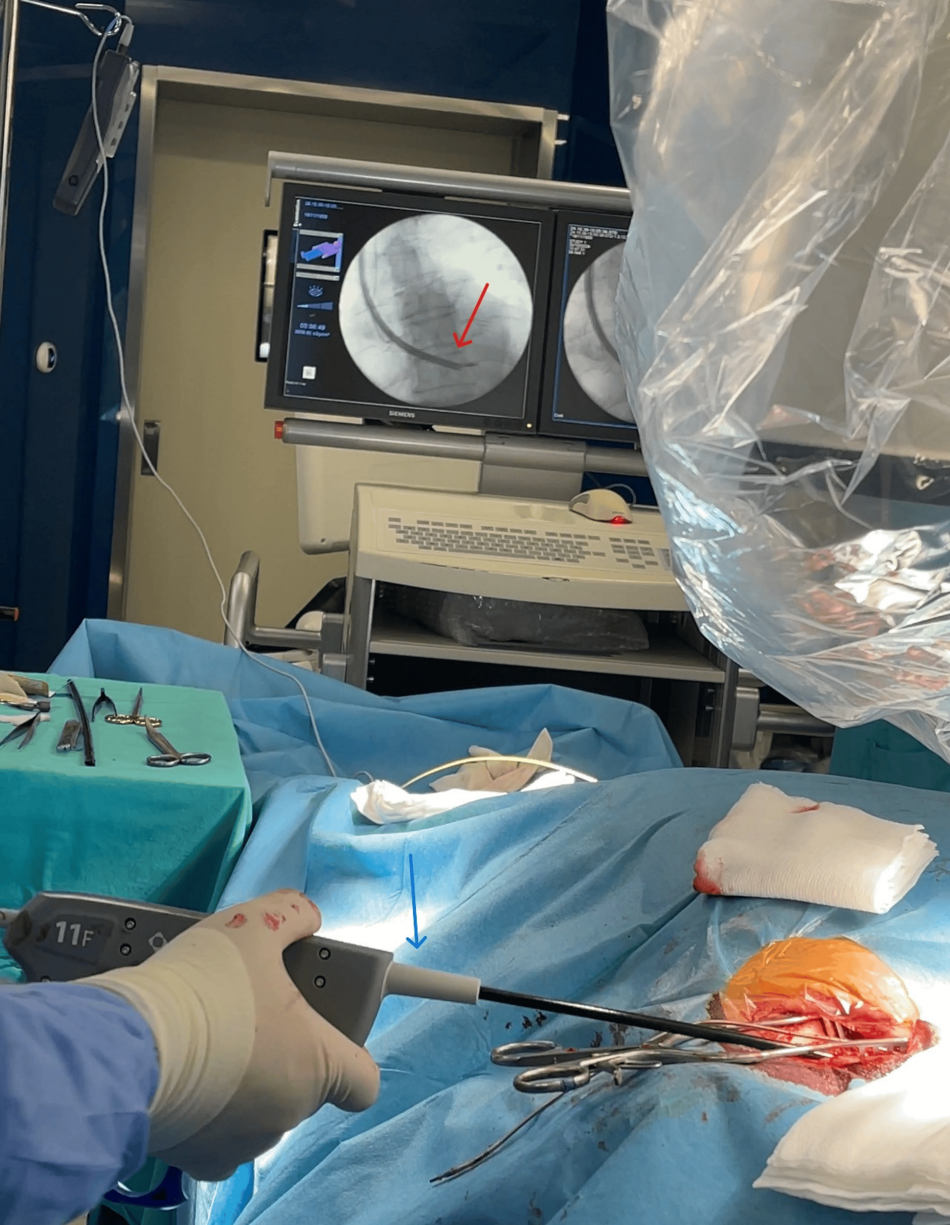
Lead extraction procedure Red arrow: Lead extraction procedure. A chest X-ray checks the position of implanted leads. Blue arrow: Lead extractor
